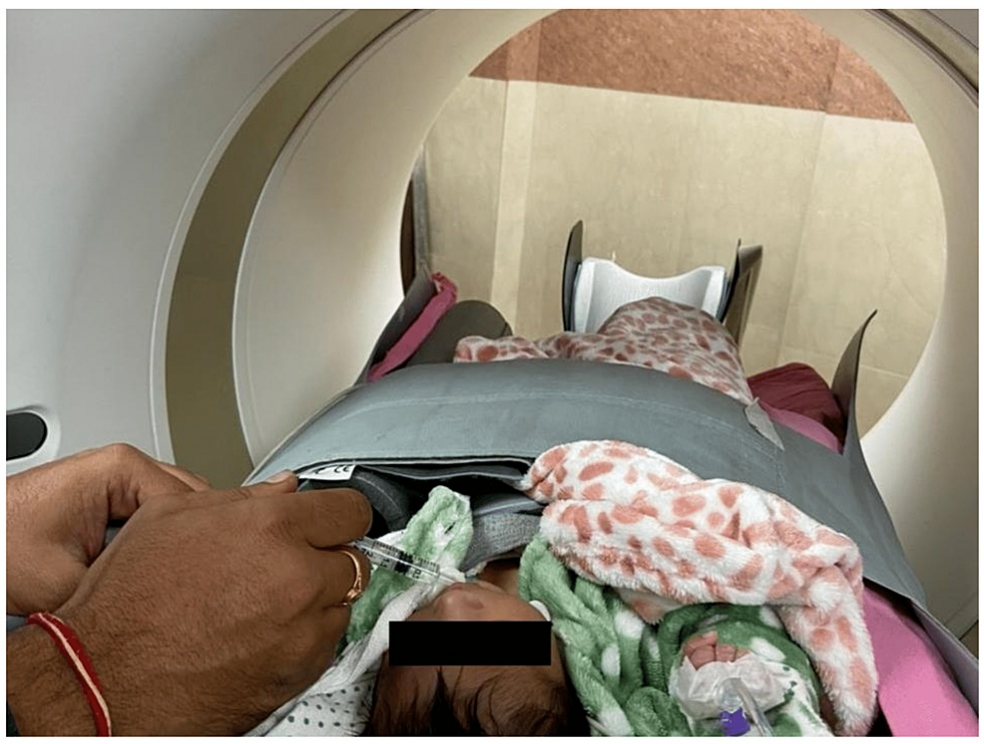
A 24-day-old neonate undergoing contrast-enhanced CT angiography using the “Feed and Wrap” technique.
